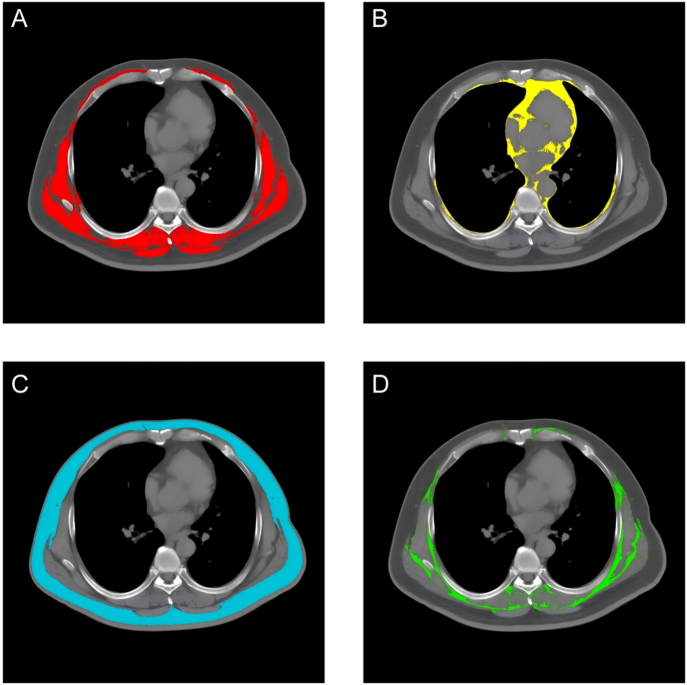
CT-based body composition measurement. This figure depicts the segmentation of an axial CT slice for quantifying body composition depots: skeletal muscle (red), VAT (yellow), subcutaneous adipose tissue (blue), and intermuscular adipose tissue (green). Detailed assessment of these compartments facilitates the study of metabolic and musculoskeletal conditions.
